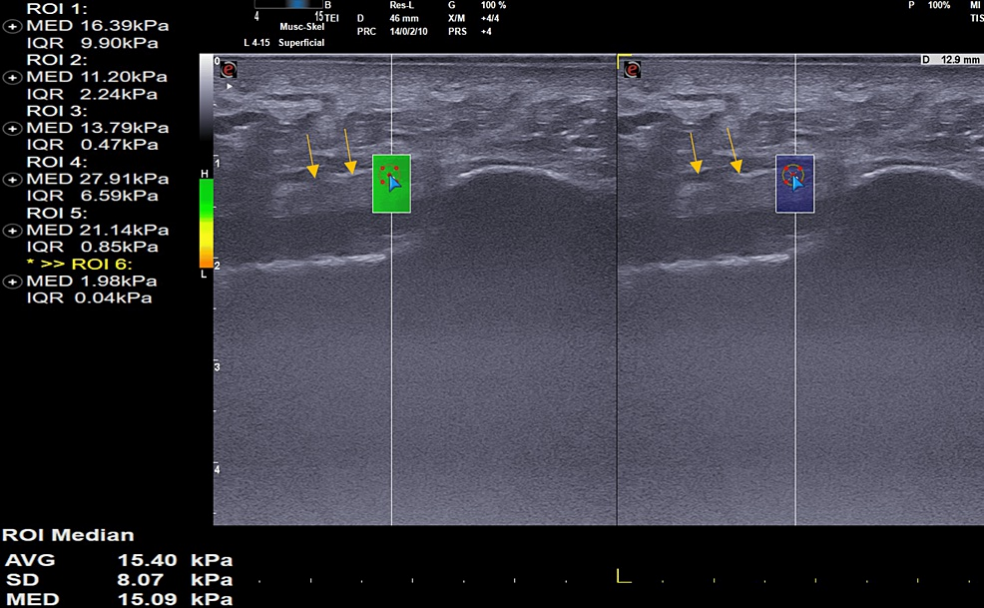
Normal plantar fascia: Sagittal B-mode ultrasound shows normal plantar fascia with the maintained fibrillar pattern (arrow showing the region of interest). The longitudinal SWE ultrasound image of a 40-year-old healthy volunteer with normal plantar fascia demonstrates a stiffness of 15.09 Kpa. SWE: Shear wave elasticity; Kpa: Kilo Pascals
